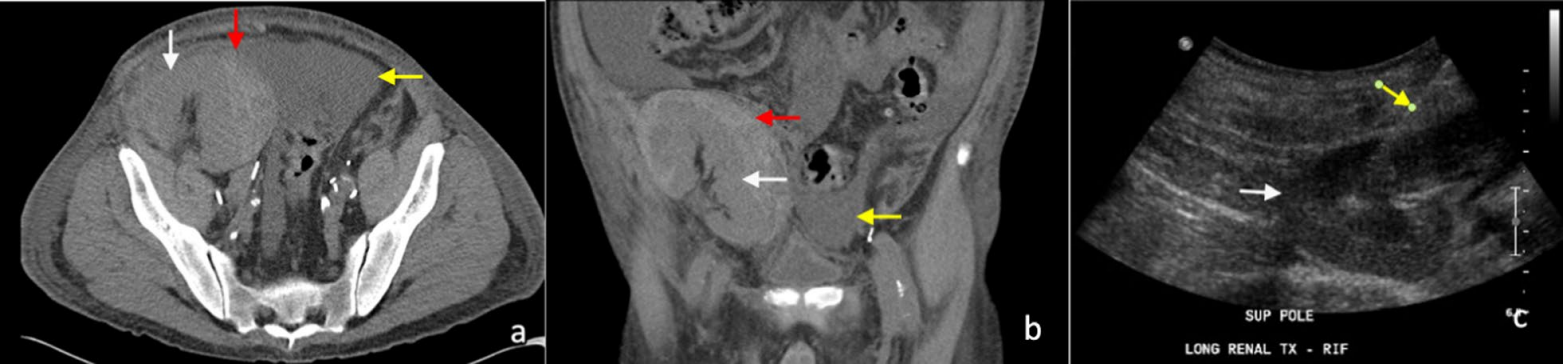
Unenhanced (a) axial and (b) coronal, CT images of a subcapsular haematoma (red arrow) following an ultrasound-guided non-targeted 14-gauge core biopsy of a right iliac fossa renal transplant allograft (white arrow). The patient had a moderate volume of ascites (yellow arrow) present prior to the biopsy. c An ultrasound-guided non-targeted core biopsy of the right iliac fossa renal graft (white arrow) was performed with a 14-gauge needle (yellow arrow) for deteriorating renal function. The single-pass 14-gauge core biopsy demonstrated features of acute on chronic graft rejection
